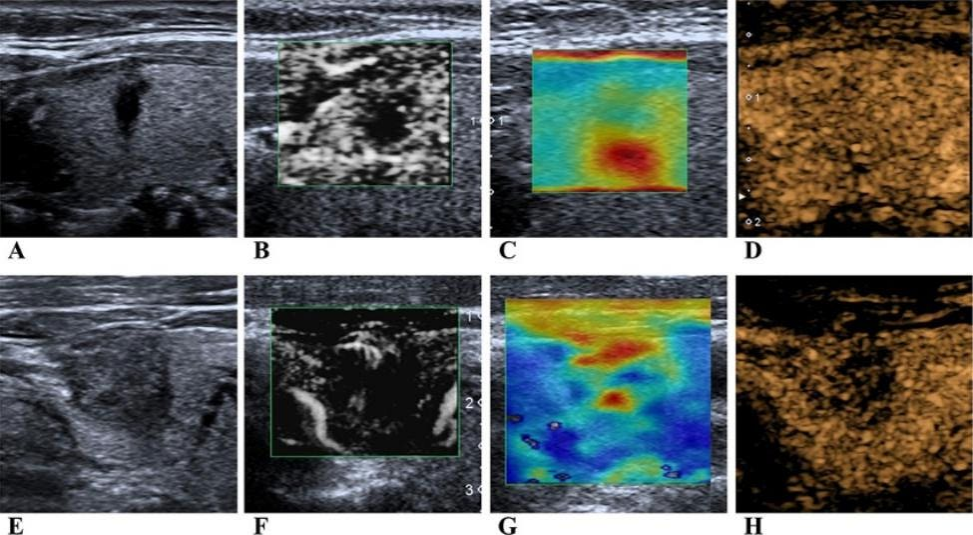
A ~ D, multimodal ultrasound images for a non-ETE tumour misdiagnosed by the multimodal model but correctly diagnosed by the clinical model; E ~ H, an ETE case misdiagnosed by clinical models but diagnosed correctly by radiomics
